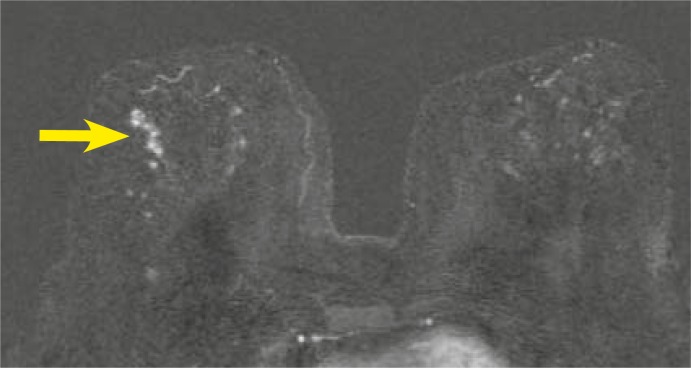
57-year old woman, US-guided fine needle biopsy performed at another institution was suspicious for pappilary carcinoma. Mammographically dense breast, first and second look ultrasound images at institute of oncology showed simple cyst. Axial T1-weighted subtracted image after. Gadolinium injection (2^nd^ minute) shows ductal, reticular-dendritic, asymmetric, non-mass like enhancement, fast initial enhancement with post initial plateau. Intermediate signal intensity on T2-weighted images. BI-RADS 4. MRI-guided vacuum-assisted biopsy revealed invasive tubular carcinoma.
